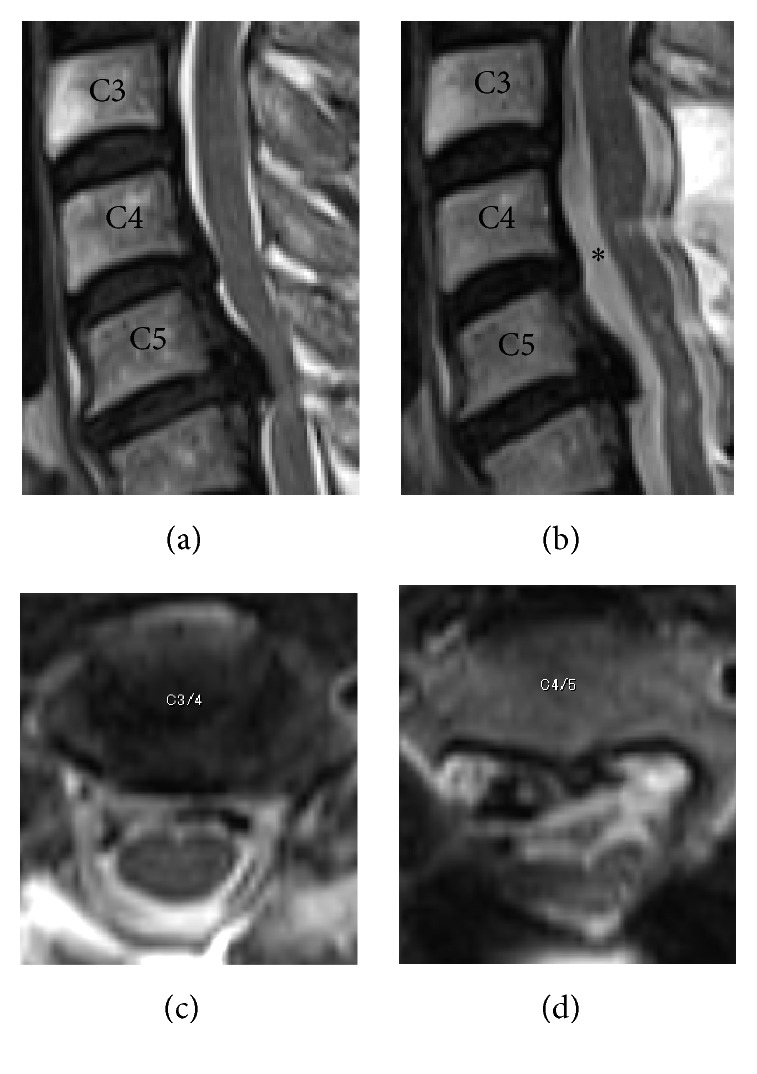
Pre- and postoperative magnetic resonance imaging. (a) and (b): posterior shift of the spinal cord at C4/5 (*∗*) was 3.8 mm. (c) and (d): sufficient decompression of the spinal cord was achieved and high-intensity areas in the spinal cord at C3/4 were not detected.
